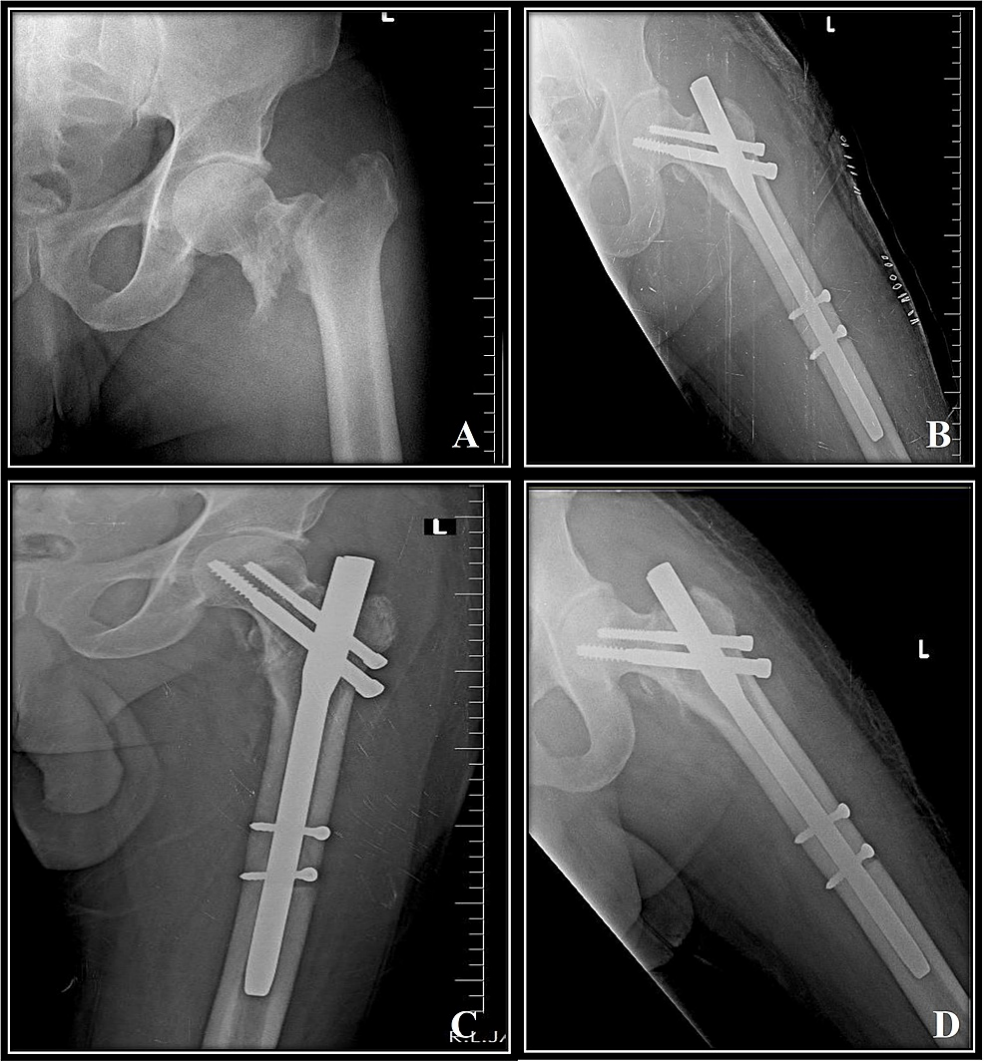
Pre-operative and post-operative follow-up radiographs of an intertrochanteric fracture treated with PFN fixation (A) Pre-operative radiograph. (B) Immediate post-operative radiograph. (C) 12-week follow-up radiograph. (D) 24-week follow-up radiograph. PFN = Proximal femoral nail
